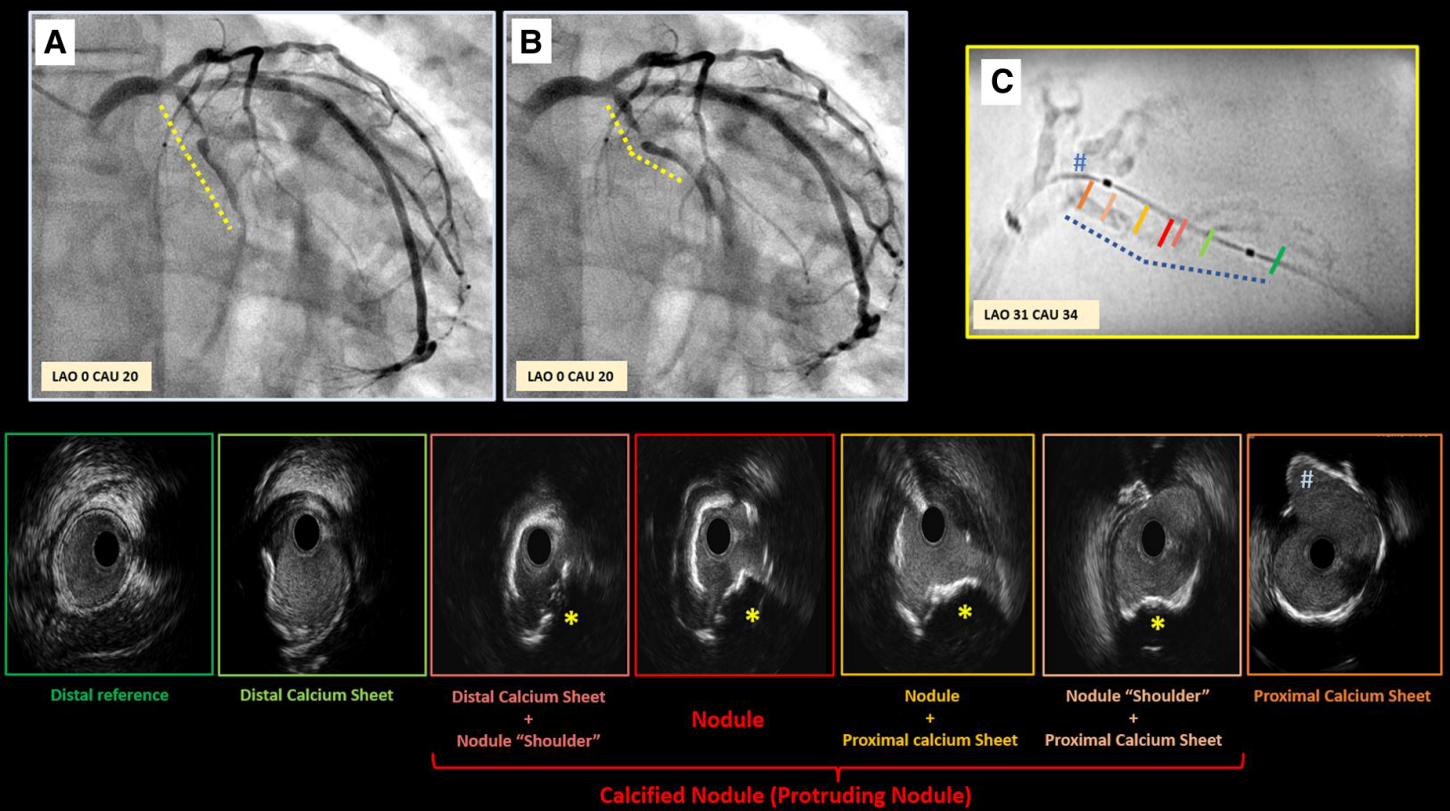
Case example supporting pathogenesis of calcified nodule. Panel A,B depict critical stenosis in left circumflex, with dotted lines highlighting the high motion and excursion of the mid part of the vessel compared to proximal segment during the cardiac cycle (Panel A exhibits diastole; B exhibits systole) with a hinge point right at the site of focal critical stenosis. Enhanced angiography clearly depicts the significant amount of calcium (Panel C). Intravascular ultrasound was performed revealing the presence of a non-concentric sheet of calcium distally that become more concentric as it gets closer to the focal stenosis (red panel) where a protruding nodule is clearly detectable (yellow star). This calcified sheet continues proximally into a non-concentric sheet of thick calcium as approaching the ostium of the first marginal branch (#).
